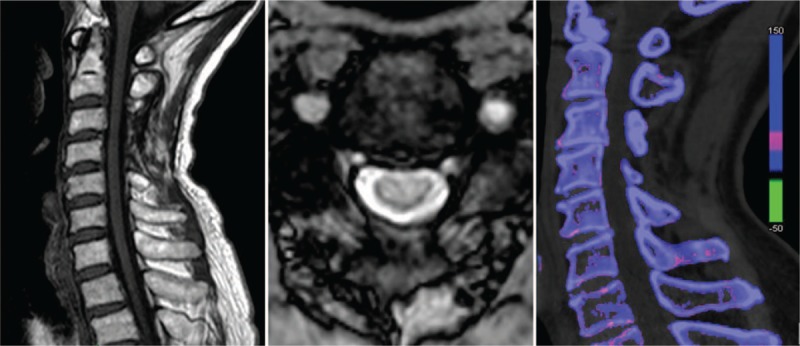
Fifth year follow-up imaging. (a, b) Axial and sagittal MRI showed complete decompression of the cervical spinal cord. (c) Dual-energy CT showed no gout deposits appeared in the cervical spine, as green represents gout.
